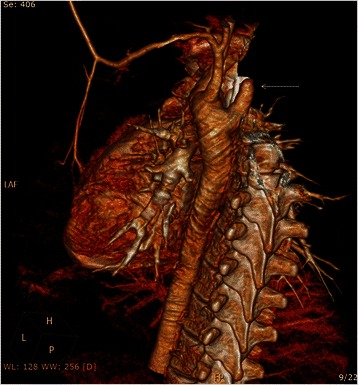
Postoperative CT angiography showing a remaining stump of the origin of ARSA
